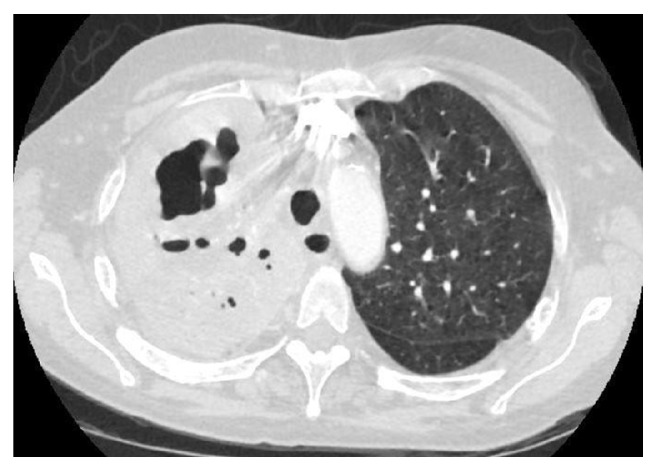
CT scan of the right upper lobe.
